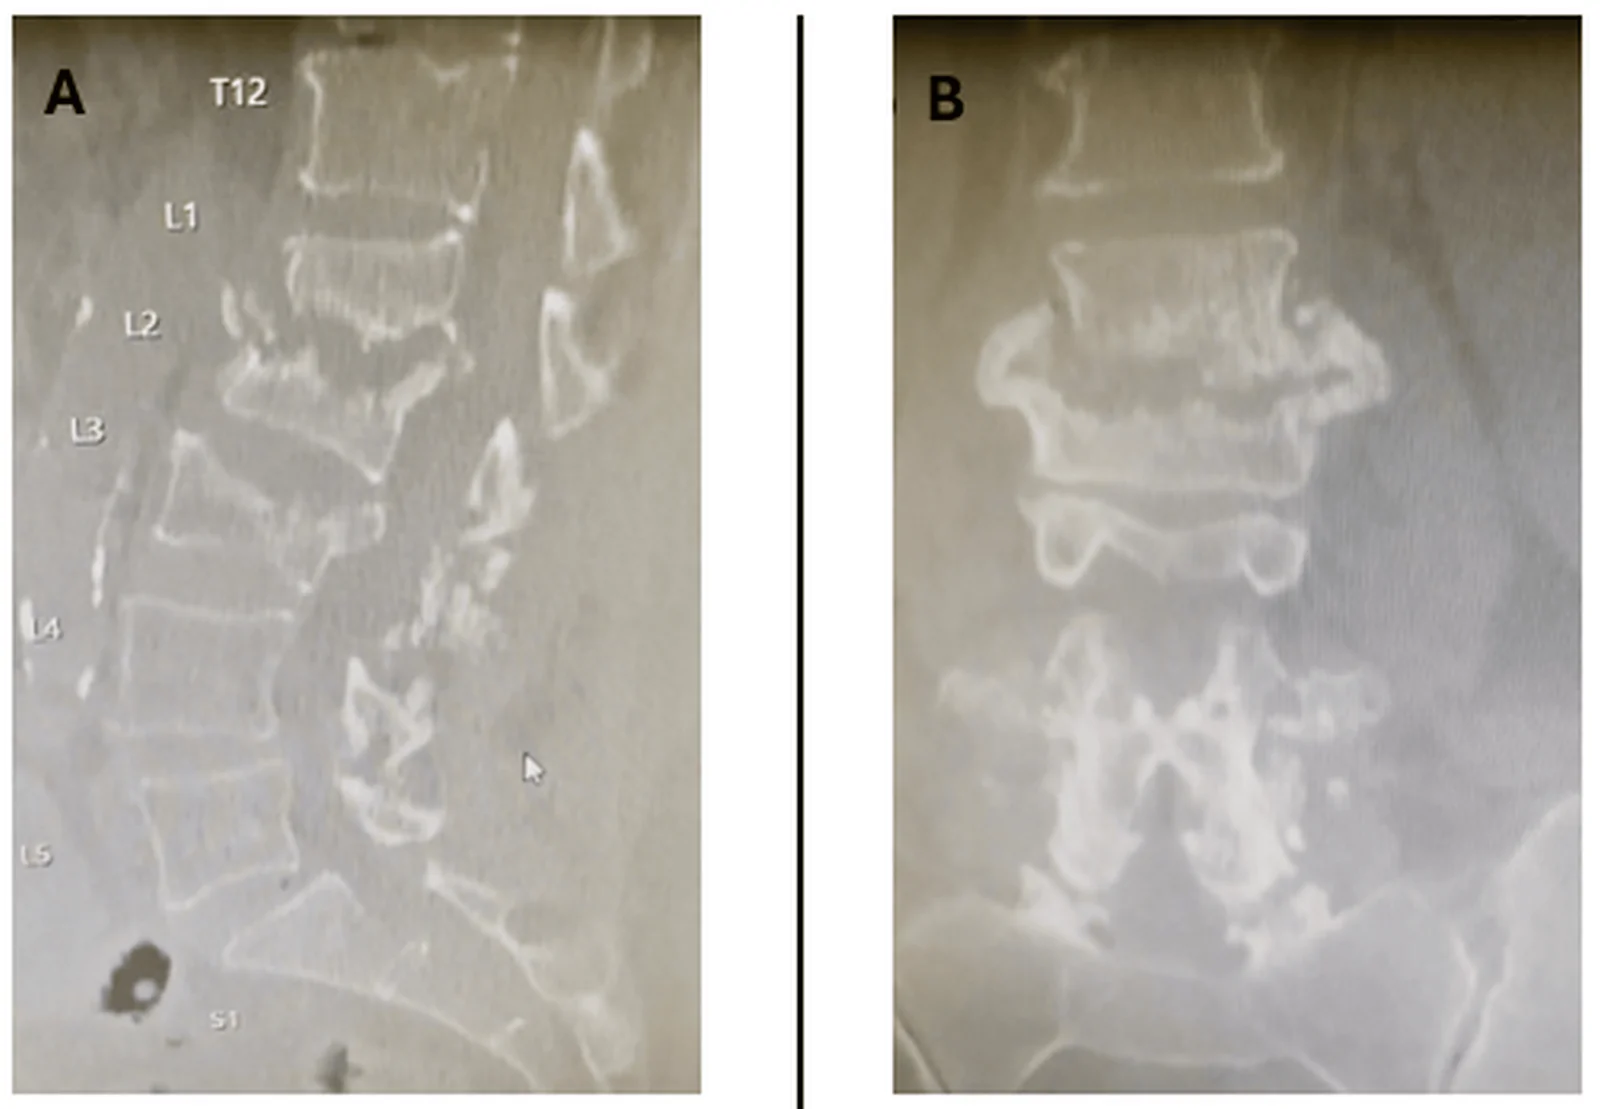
(A) Preoperative sagittal CT of the lumbar spine demonstrating extensive bony destruction at L1 and L2 with endplate irregularity, consistent with persistent osteomyelitis and discitis, (B) Preoperative coronal CT of the lumbar spine confirming extensive bony destruction of the same segment.
